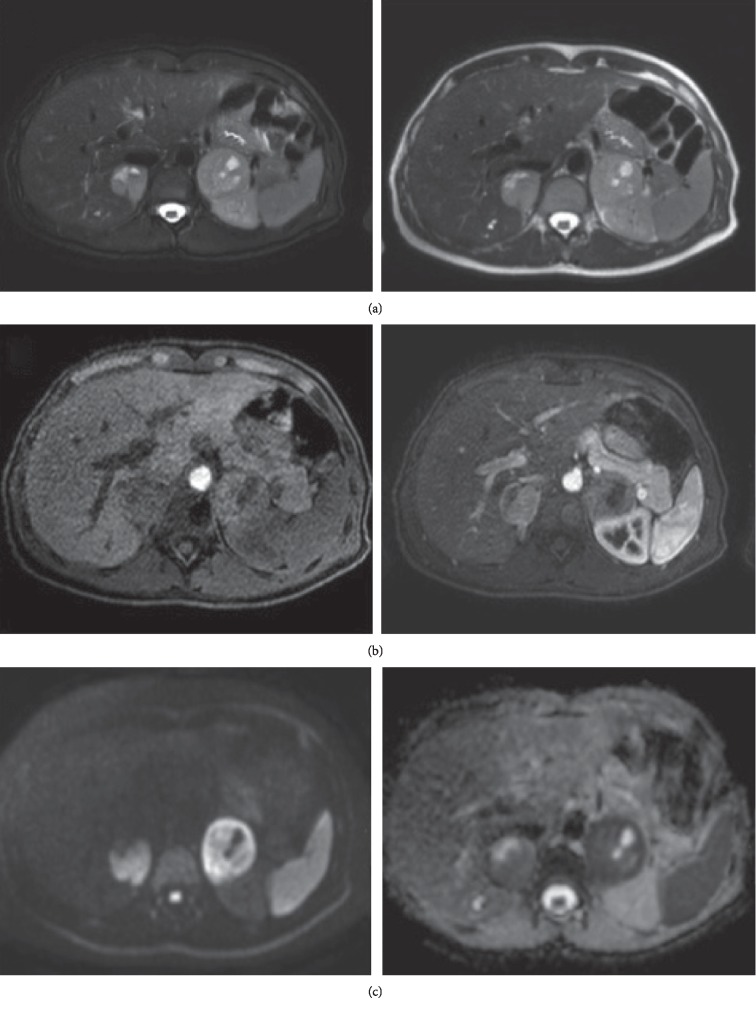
Abdominal MRI scan showing bilateral phaeochromocytoma. (a) axial TSE-T2W images with and without fat suppression; (b) axial GRE-T1W images prior to and 35 s after contrast injection; (c) DWI and ADC map showing restricted diffusion (ADC = 0.6 × 10^−3^ mm^2^/s).
